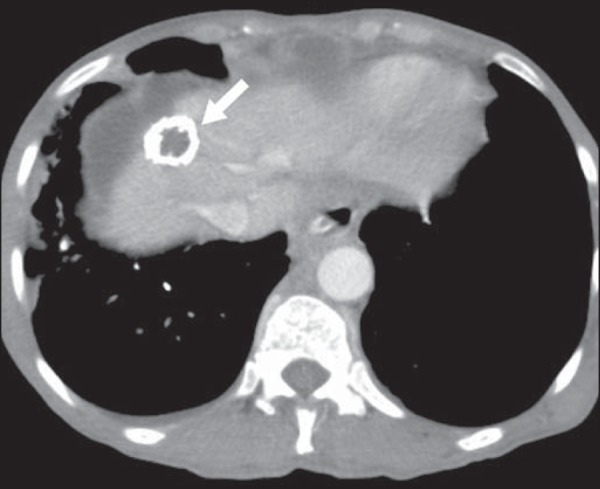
Blue rubber bleb nevus syndrome. Axial CT scan showing a calcified
